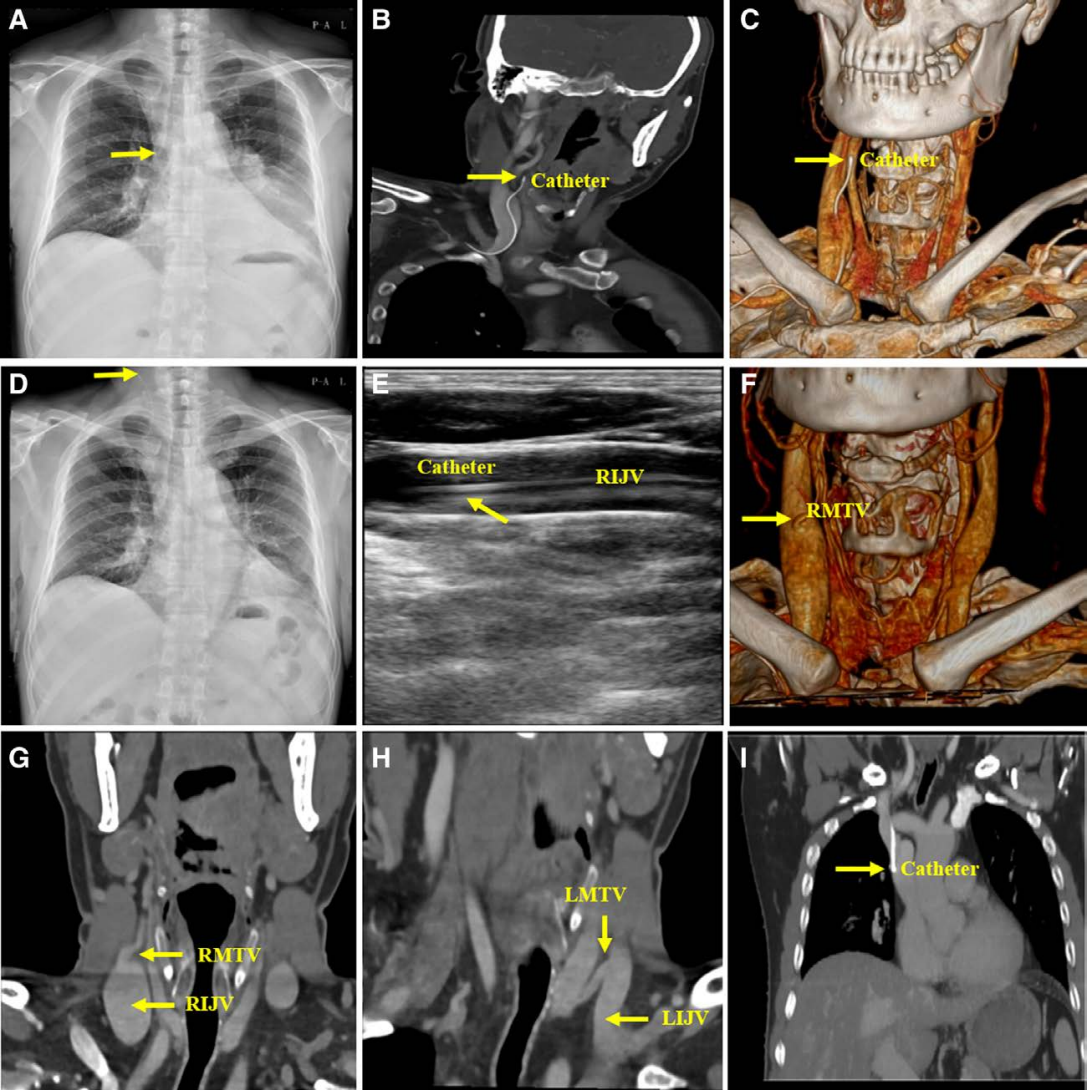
Radiographic information for Patient 2.
